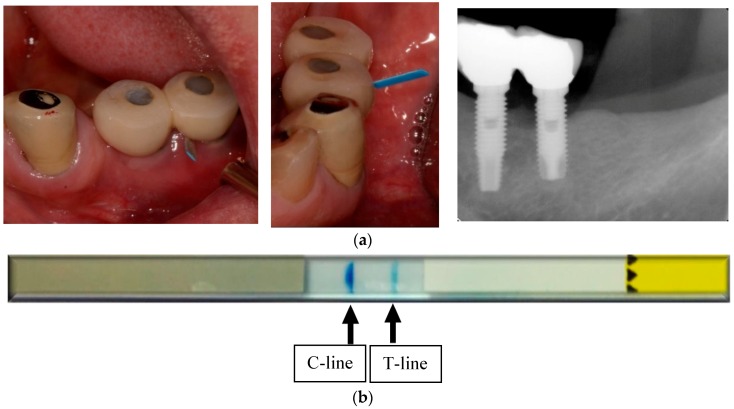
(a) Demonstrate the clinical and radiographic features of peri-implantitis and use of ImplantSafe; (b) Two clear lines indicate elevated risk for peri-implantitis. A positive test result: both control (C) line and test (T) line are visible, reveals elevated collagenolytic activity.
